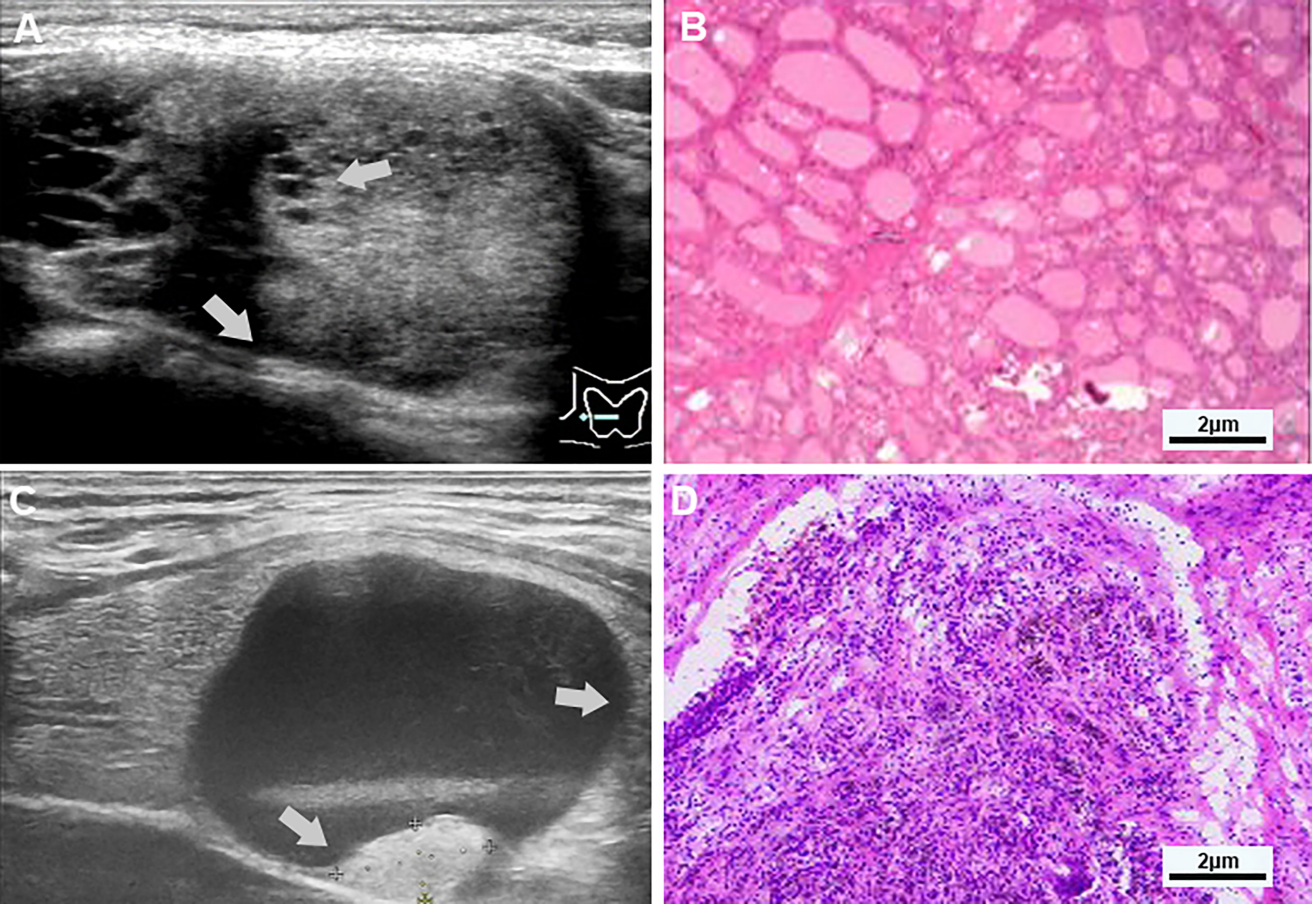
(A, B) Examples of typical ultrasonographic and pathological sections of multiple separation partially cystic thyroid nodules. Transverse section of a cystic-solid nodule of the thyroid, mainly solid, with smooth and irregular margins, and an acute angle between the multiple-separated and fused solid components and the nodule wall (indicated by gray-white arrows), for the hypoechoic, the free edge is non-smooth, and the pathology confirmed nodular goiter. (C, D) Examples of typical ultrasonographic and pathological sections of predominant cystic partially cystic thyroid nodules. Longitudinal section of a cystic-solid nodule of the thyroid, mainly cystic, with smooth, oval nodule margins, and an obtuse angle between the solid part attached to the posterior wall and the nodule wall (indicated by the gray-white arrow), For isoechoic, the free edge is smooth, and the pathology confirmed nodular goiter with cystic degeneration, close to the thyroid membrane.
